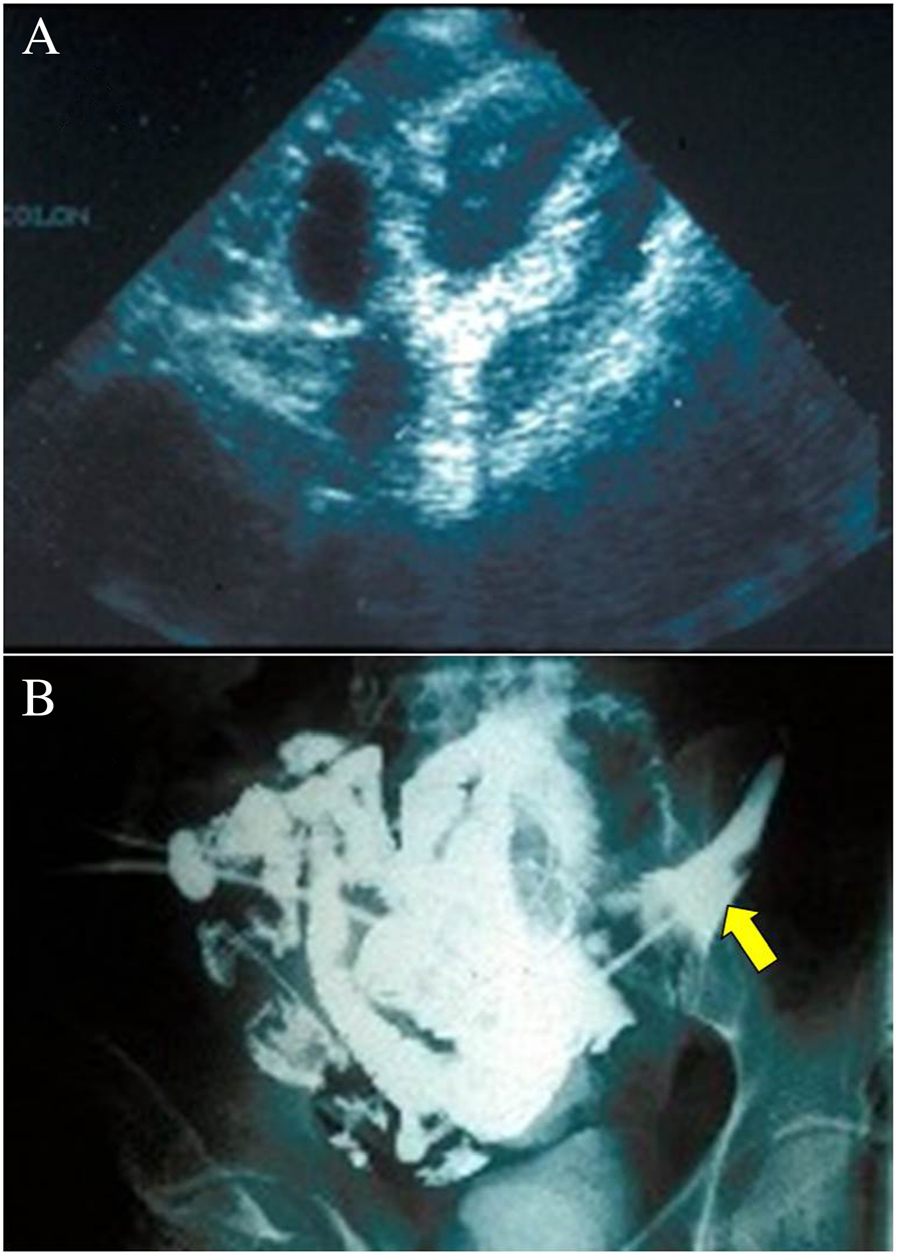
A 50-year-old man presented with abdominal pain of 1 year duration and weight loss. Abdominal examination revealed an abdominal mass in the left lower quadrant. The patient had anemia (hemoglobin of 87 gm/L) and hypoalbuminemia (28 g/L). Abdominal ultrasound (a) showed matted bowel loops, thickened mesentery, and presence of intraperitoneal fluid. CT abdomen showed thickened intestine with localized ascites and retroperitoneal small lymph nodes. Diagnostic laparoscopy was tried to harvest a biopsy (b). It was difficult and a perforation of the small bowel was suspected. Laparotomy was performed which showed that the small bowel was matted. Intraoperative frozen section confirmed the diagnosis of abdominal tuberculosis. Two iatrogenic small bowel perforations were closed using absorbable sutures. The patient developed postoperative small bowel fistula (yellow arrow)
